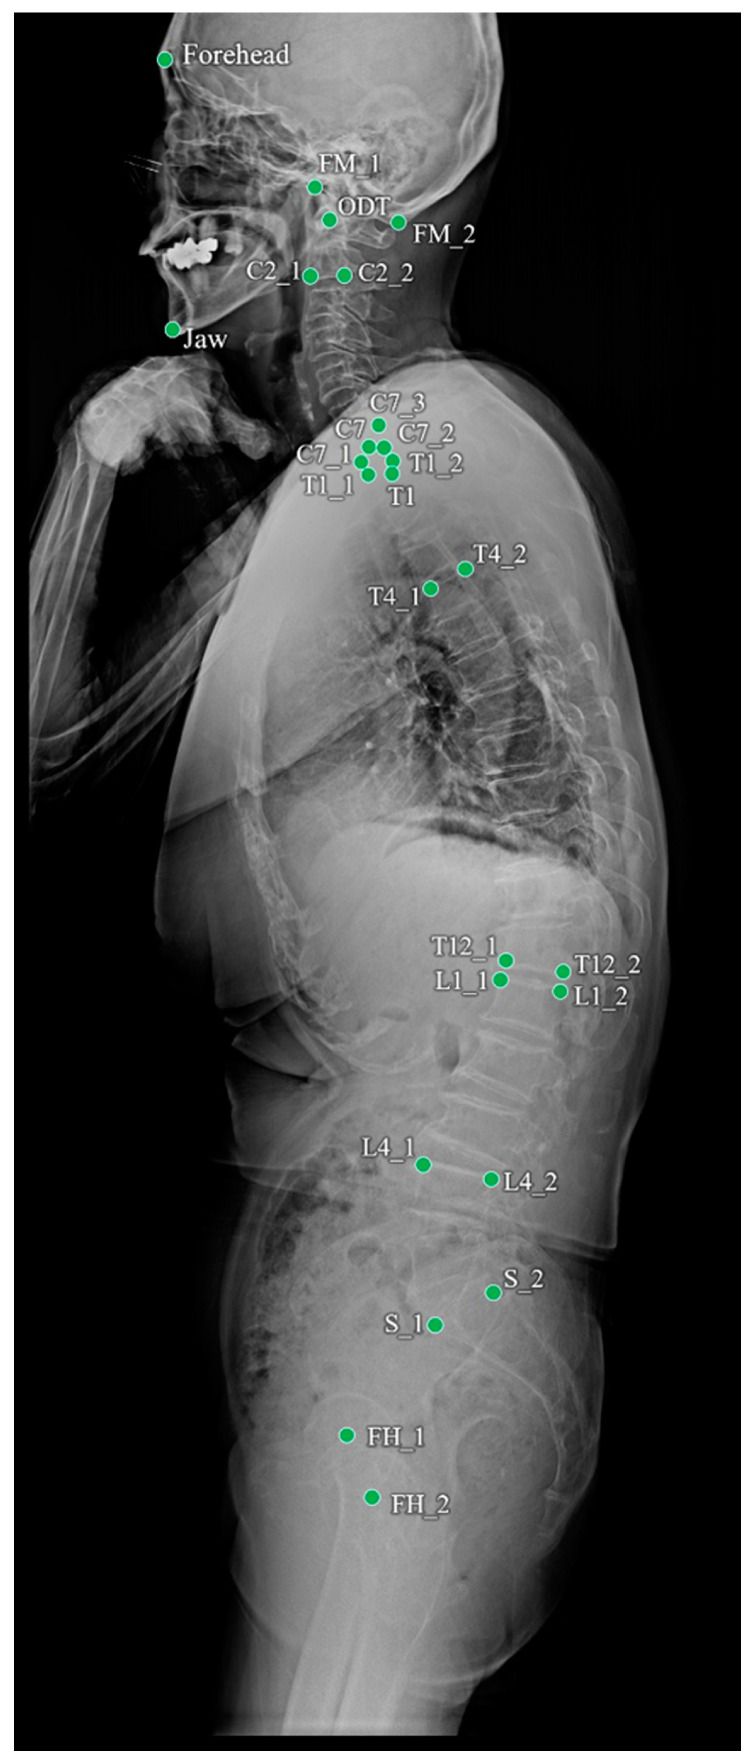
Landmarks annotated on a whole-spine lateral radiograph.
